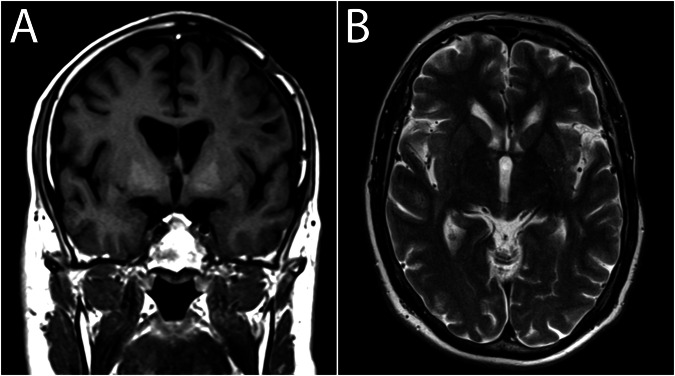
The effect of Gd–ferritin interactions. A non-contrast T1-weighted image displays visibly increased signal intensity in the globi pallidi. B T2-weighted image shows physiological hypointensity in the globi pallidi. Additional T2 shortening due to the Gd–ferritin interactions cannot be appreciated by the naked eye
